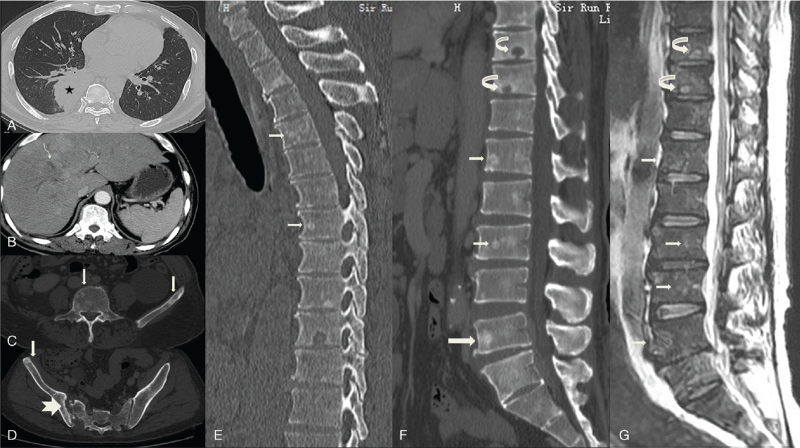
(A) Soft tissue mass in the right lower lung (black star). (B) A low-density mass (thin curved arrow) can be seen near the hepatic hilum, accompanied by distant bile duct dilatation. (C-F) Multiple sclerotic foci can be seen in the thoracolumbar spine and ilia: some small circular high-density lesions (thin straight arrow), partly agglomerated high-density lesions with an ill-defined margin (thick straight arrow), and partial round destruction of the endplates with a sclerotic margin (curved arrow). (D) Note the destruction of bone on the right sacroiliac articular surface, with irregular and sclerotic margins (swallow tail arrow). (G) Sagittal T2-weighted imaging shows high signal intensity of the lesion (thin straight arrow), high signal intensity of the rounded lesions at the endplate with low signal margins (curved arrow), and no signal reduction or other degenerative manifestations in the adjacent intervertebral disk.
